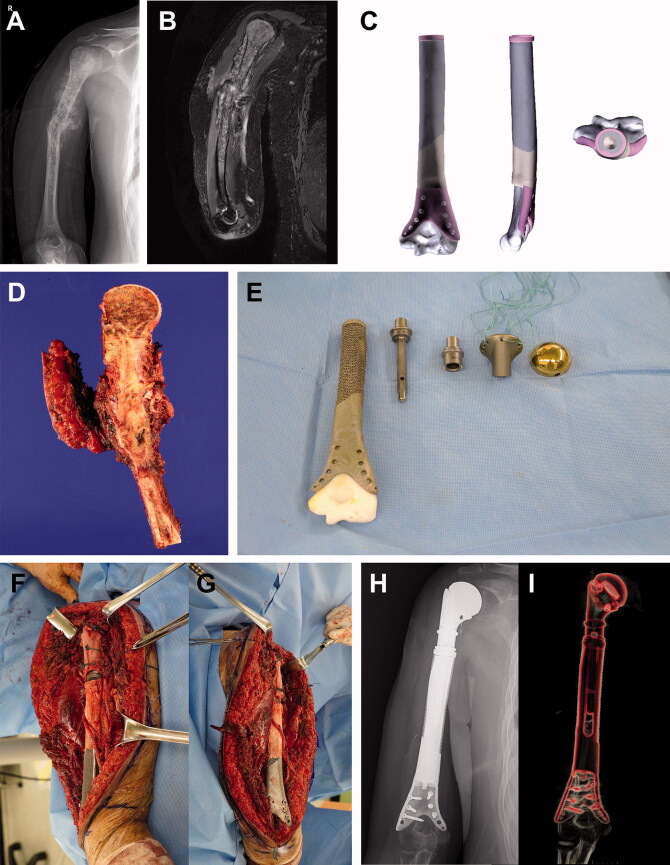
Humeral reconstruction. Images of patient #10. (A) A preoperative plain radiograph and (B) a T2-weighted MR image showing osteosarcoma in most of the humerus, except the elbow joint. (C) Graphical designs of the 3D-printed implant. (D) Resected bone tumor as planned. (E) Photograph of the 3D-printed implant and tumor prosthesis to be used. Intraoperative photograph showing (F) proximal and (G) distal parts of the reconstruction. (H) A postoperative plain radiograph and (I) CT reconstruction image showing humeral reconstruction.
